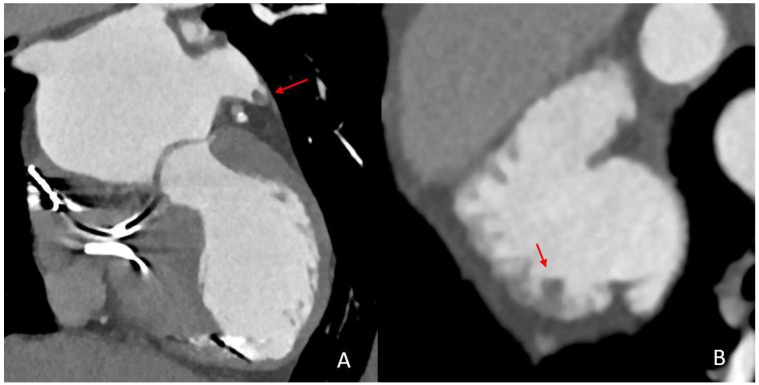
Thrombus in the cauliflower-shaped LAA. In this patient with LVO, a persistent thrombus was depicted on cCT in the framework of stroke etiology assessment (red arrow, image (A)). The LAA exhibits a distinct cauliflower shape with multiple small indentations, which can be delineated in multiplanar reconstructions on the CT imaging (red arrow, image (B)).
